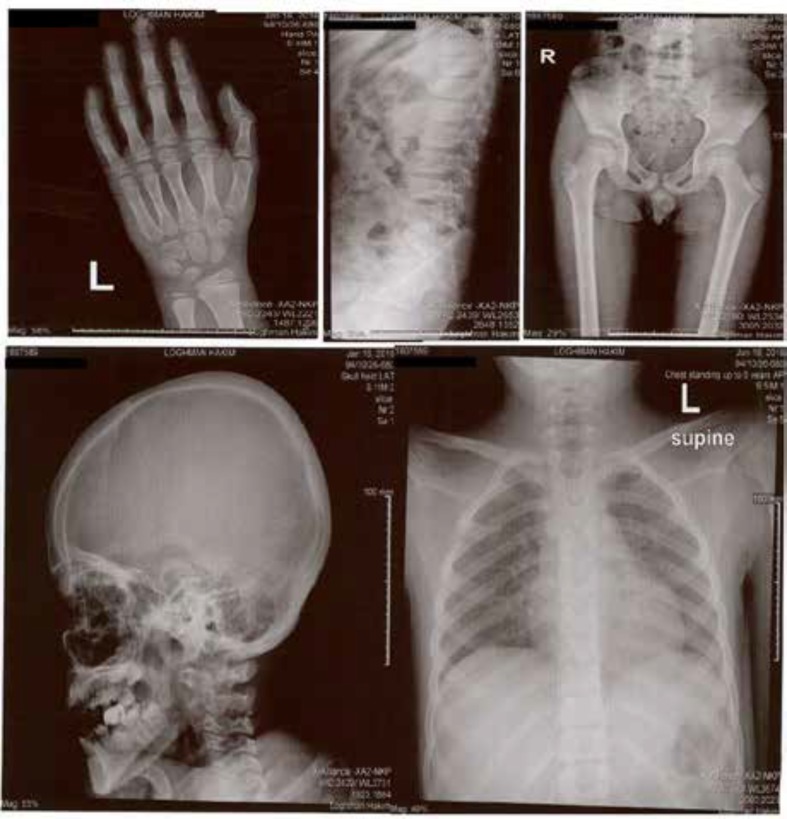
Radiographic evaluation of hand and wrist, lumbar spine, pelvis, head and neck and thorax
